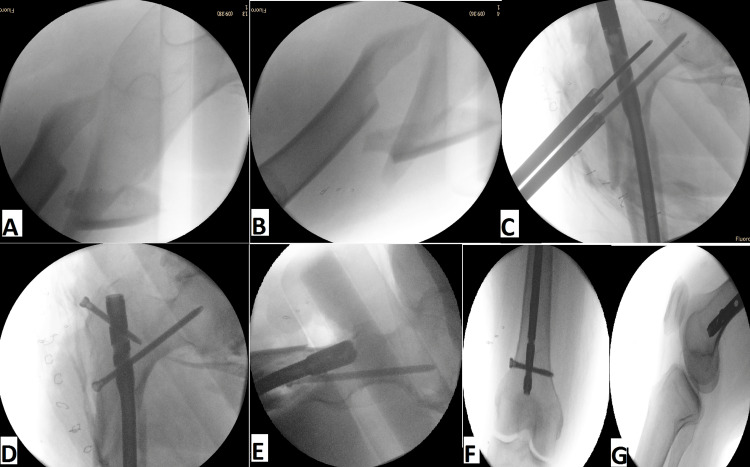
Intraoperative anteroposterior and lateral radiographs (A, B) of the proximal femur show a severely displaced subtrochanteric femur fracture. Anteroposterior and lateral radiographs (C, D, E, F, G) of the left femur show the intraoperative steps of intramedullary nail insertion.
